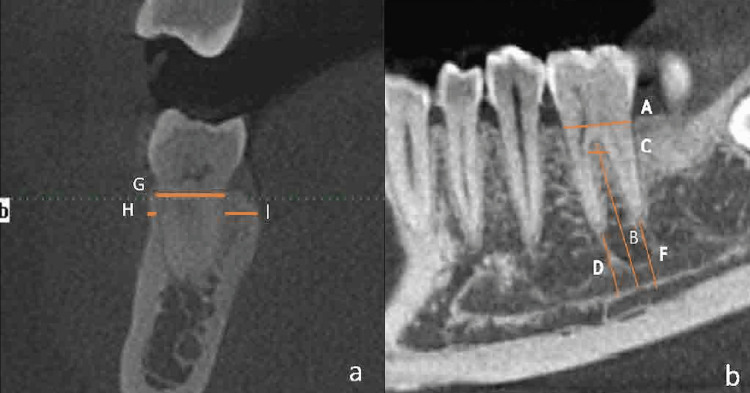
Mandibular molar measurement CBCT scan a: Coronal section. b: Sagittal section. A: Mesiodistal socket size. B: Distance from IAN to the furcation. C: Width of the interradicular bone 2 mm apical to the furcation. D: Distance from the IAN to the apex of the mesial root. F: Distance from the IAN to the apex of the distal root. G: Buccolingual socket size. H: Width of the buccal bone. I: Width of the lingual bone
